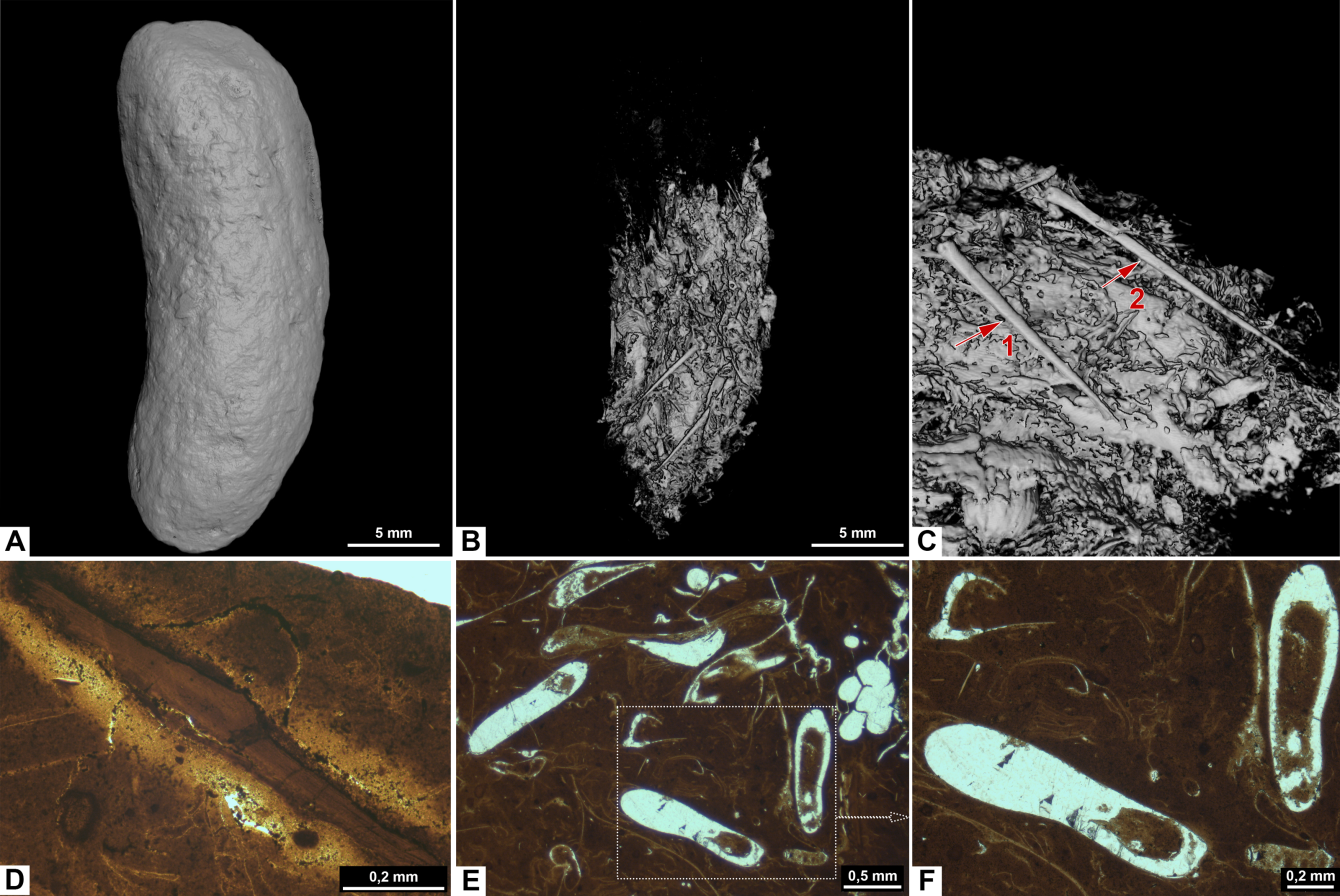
CT-scans (A–C) and thin sections (D–F) of morphotype D. (A) External view of morphotype D, PMO 250.528. (B–C) Internal view of PMO 250.528 Red arrows pointing to bone inclusions with arrow 1 measuring 3.6 mm and arrow 2 measuring 5.4 mm. (D) Bone fragment, PMO 250.005. (E–F) Mineralized inclusions and possible onychites, PMO 250.005.
